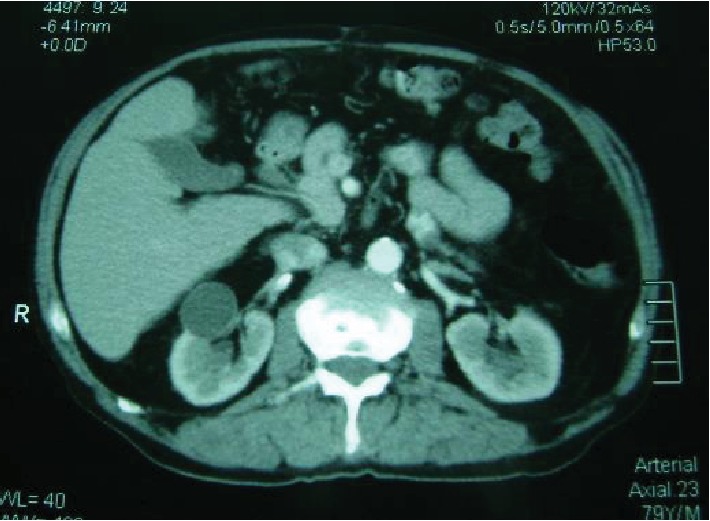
Axial CT image of the abdomen reveals a lesion involving the right kidney cortex and parenchyma.
